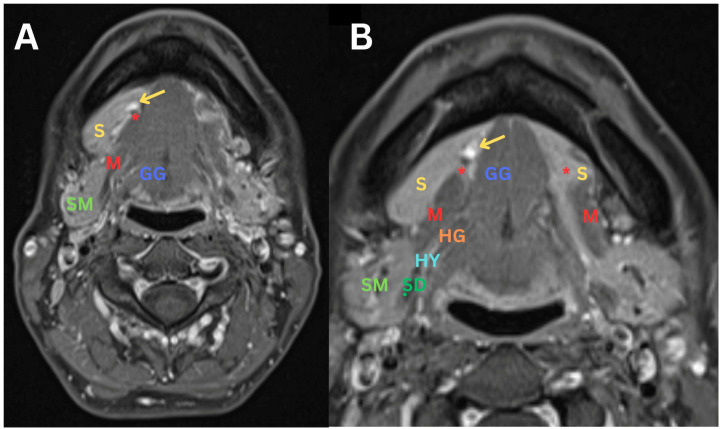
(A) An MRI T1 TSE Dixon axial sequence showing a defect in the right mylohyoid muscle (M), accompanied by the protrusion of the right sublingual salivary gland (S). (B) A closer view using the same T1 TSE Dixon axial sequence, highlighting the protrusion of the right sublingual salivary gland (S), with several small sialoceles indicated by an arrow in the background, suggesting a chronic process. Sialoceles occur in the context of mylohyoid boutonnieres due to the displacement of the sublingual gland through a defect or gap in the mylohyoid muscle. This anatomical variation leads to the accumulation of saliva in the surrounding tissue, as the herniated gland may become obstructed or damaged. The mylohyoid muscle typically supports the sublingual gland, and when a boutonniere defect is present, the muscle’s integrity is compromised, allowing the gland to prolapse and potentially disrupt normal salivary drainage. As a result, saliva can collect in the tissues, forming a sialocele. This condition highlights the importance of understanding the anatomical relationships and variations in the submandibular region to avoid misdiagnosis and inappropriate management. Similar but less pronounced changes are observed on the left side, where there is a minor protrusion of the left sublingual salivary gland (S). It is notable that the signal intensity of both sublingual salivary glands (S) is similar, and the contrast is homogeneous, with no MRI findings suggesting malignancy. S—sublingual gland; M—mylohyoid muscle; *—mylohyoid muscle defect; GG—genioglossus muscle; SM—submandibular gland; SD—submandibular duct (also known as Wharton’s duct); HG—hyoglossus muscle; HY—hypoglossal nerve.
